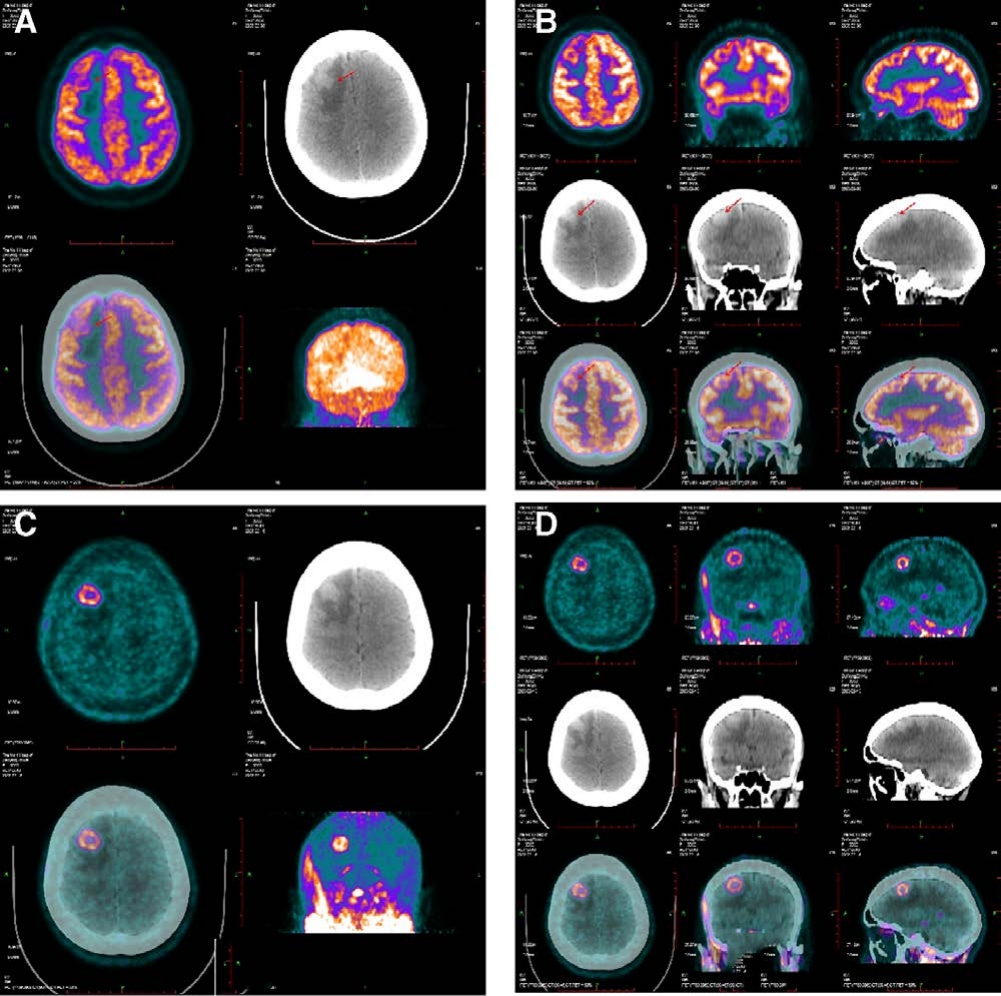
^18^F-FDG and ^11^C-CHO PET/CT imaging (A) ^18^F-FDG PET/CT imaging, (B) ^18^F-FDG PET/CT imaging, (C) ^11^C-CHO PET/CT imaging, (D)^11^C-CHO PET/CT imaging. ^18^F-FDG = ^18^F-fludeoxyglucose, PET/CT = positron emission tomography/computed tomography.
